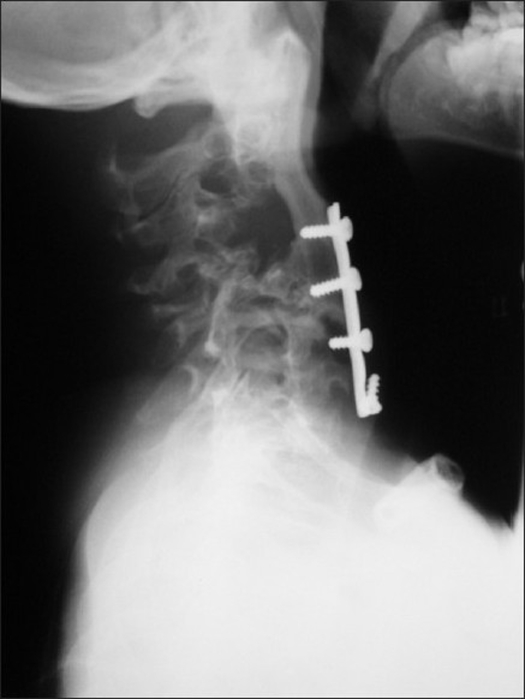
The radiograph shows the lower 2 screws were loosened during the growth of the lower cervical spines (C6 and C7)
